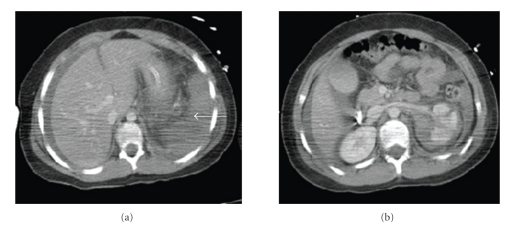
Followup CT scan of the abdomen in portal venous phase shows relative hypodensity of the spleen (white arrow), increase in intraperitoneal fluid, and unchanged appearance of  left kidney.
